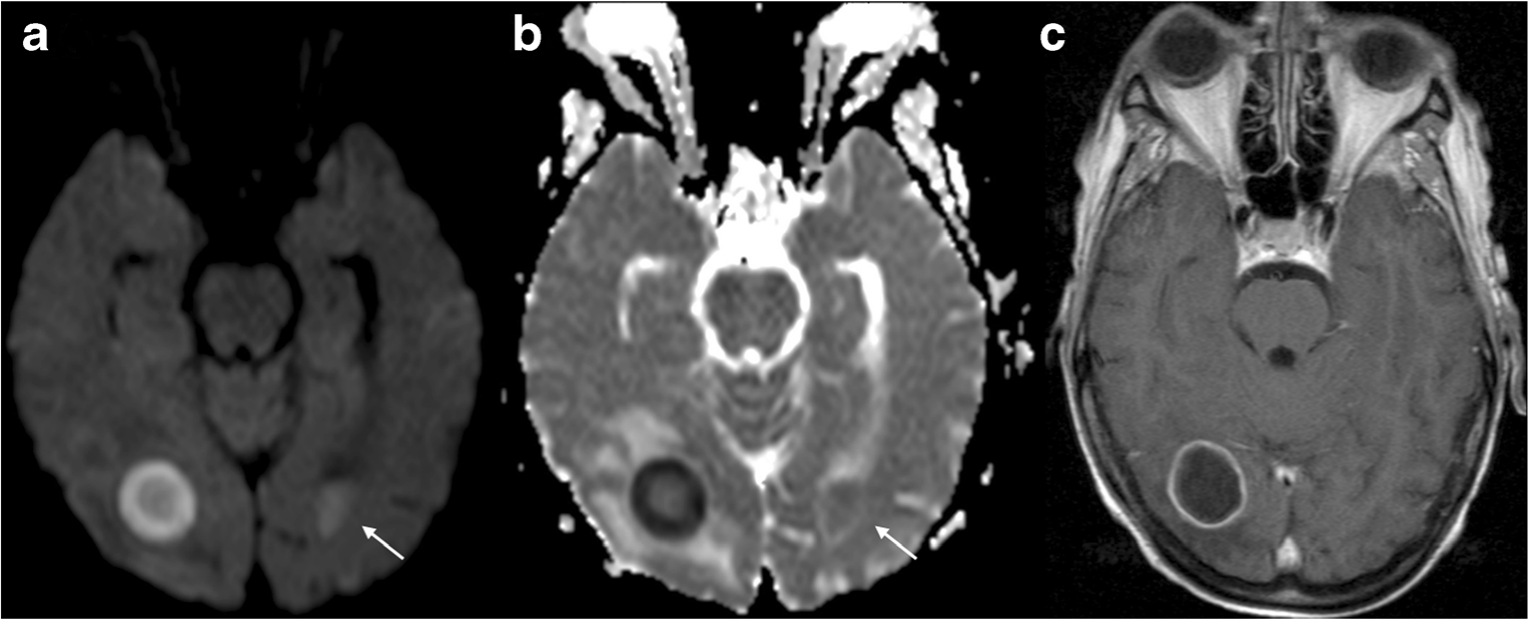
Abscess. Right occipital mass showing marked diffusion restriction within the core on DWI and ADC maps (a and b, respectively) and a peripheral enhancing pattern on T1 post gadolinium (c). DWI helps to differentiate ring-enhancing lesions because restricted diffusion in the centre of the mass is characteristic of pyogenic abscesses. In this case, diffusion-based sequences also helped to identify ventriculitis (arrows)
